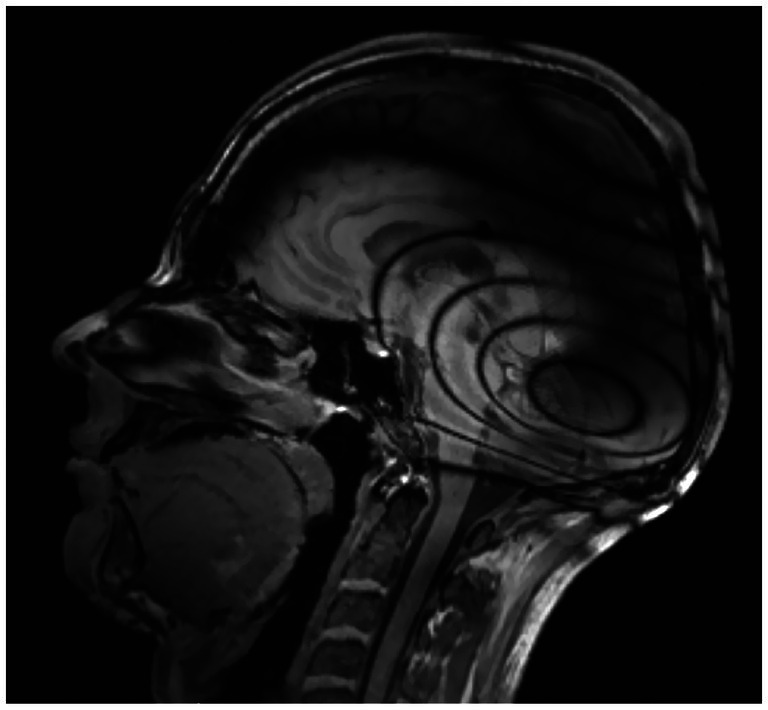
T1-weighted sequence in a bilateral CI with a magnet positioned at a 135° angle demonstrating that the nasopharynx is only partially assessable.
